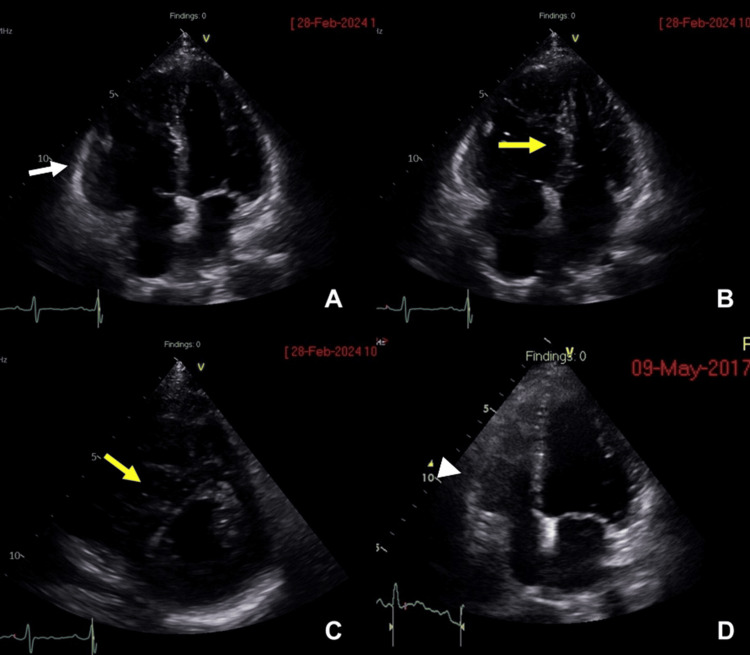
Transthoracic echocardiogram showing the dilated right ventricle An apical four-chamber right ventricle (RV) focused view (white arrow, panel A), with systolic flattening of the septum on the apical four-chamber view and parasternal mid-ventricular short-axis view (yellow arrow, panels B and C), in keeping with RV systolic pressure overload. Panel D shows normal RV dimensions with an RV basal diameter of 29 mm on apical four-chamber view (white arrowhead) on echocardiogram performed in 2017, prior to commencing treatment with trastuzumab emtansine
